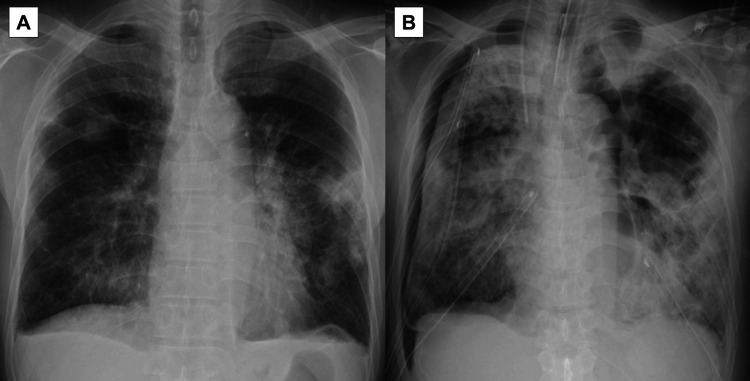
A) Chest radiography on the day of admission showed multiple nodular and infiltrating shadows in both lung fields. B) Chest radiography on the seventh day after admission showed bilateral pneumothorax
